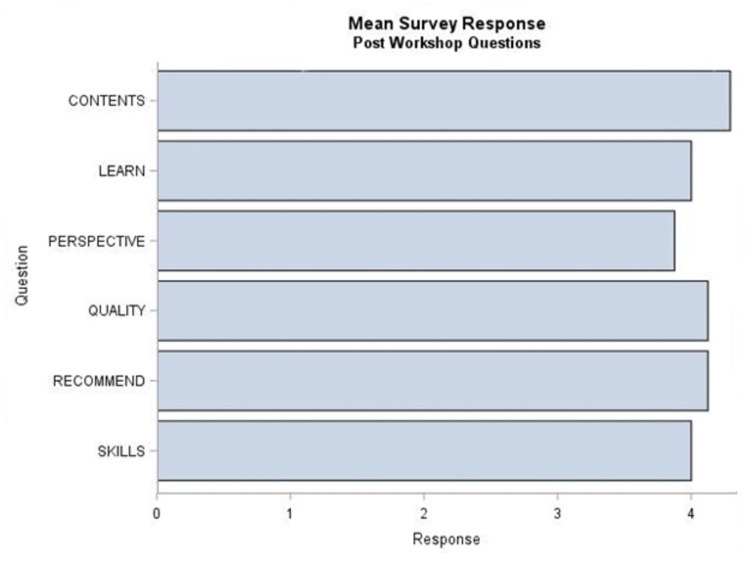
Summary of learner satisfaction with the workshop (Kirkpatrick level 1). Resident physicians rated the extent of agreement or disagreement with each statement as strongly disagree (1), disagree (2), neither agree nor disagree (3), agree (4), and strongly agree (5). CONTENTS - I am satisfied with the contents of the workshop. LEARN - This workshop encouraged me to learn more about the topic. PERSPECTIVE - This workshop changes my perspective on leadership. QUALITY - The quality of this workshop matched my expectations. RECOMMEND - I will recommend this workshop to others. SKILLS - This workshop addressed the skills I need to improve my team.
